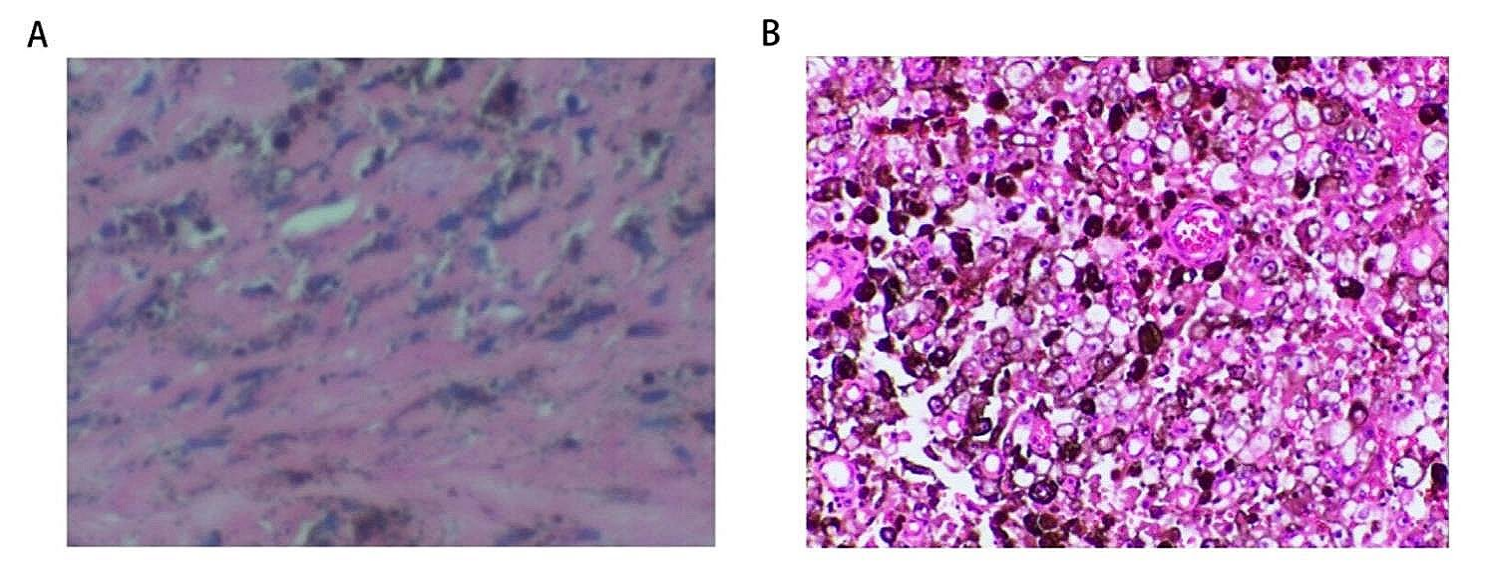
MRI of the cervical spine, showing a space-occupying lesion in the cervical spine. A. Enhancement item, coronal position; B. MRI enhancement, cervical sagittal view; C. T1 item, cervical sagittal view; D. T1 cross-section.; E. T2, cross-section. F. Shows the tissue window after surgery; G. Shows the bone window after surgery
